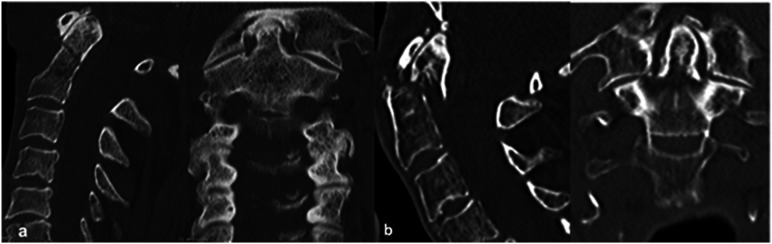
Sagittal and coronal computed tomography images of patients with type II odontoid fracture following (a) high-energy trauma and (b) low-energy trauma, depicting severe osteoporosis at the dens-body junction and no osteoarthritis in the lateral atlantoaxial joint.
